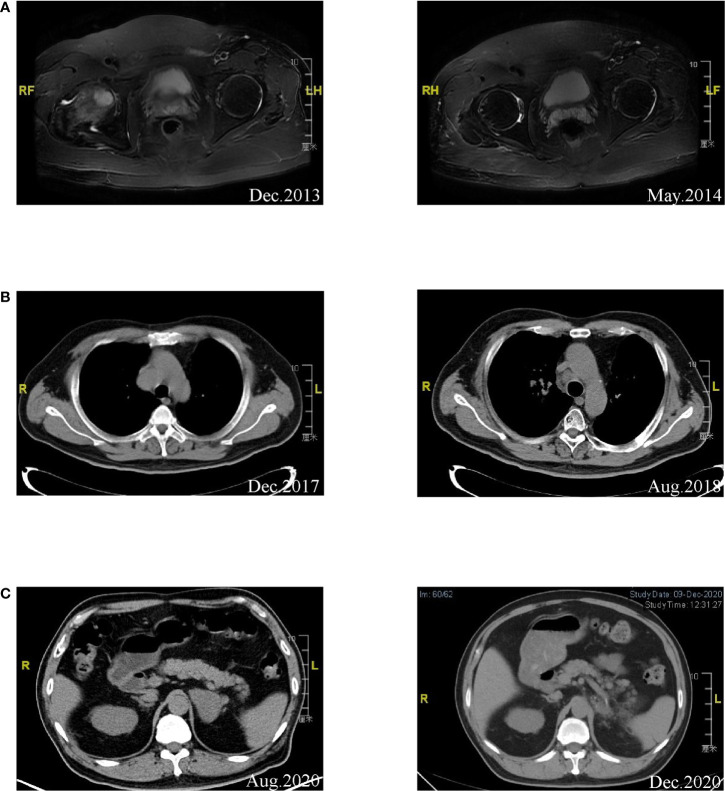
(A) Left: MRI of right femoral head indicating metastasis diagnosis. Right: A partial response was achieved after radiotherapy and gefitinib treatment. (B) Left: Disease progression was found in mediastinal lymph nodes by CT. Right: A partial response was achieved after continued gefitinib treatment and radiotherapy. (C) Left: Metastasis was detected in the left adrenal gland. Right: CT image of the patient after left adrenalectomy was performed.
